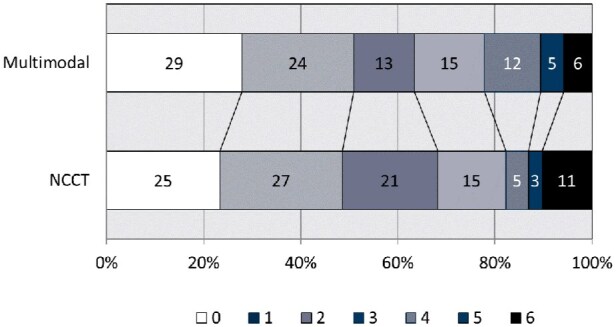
Modified Rankin scale distribution at day 90 follow-up. Adjusted odds ratio 0.93 (0.57, 1.51, *p* = 0.758). NCCT: non-contrast computed tomography; CT: computed tomography. Numbers in each section are the number of participants in each outcome category.
